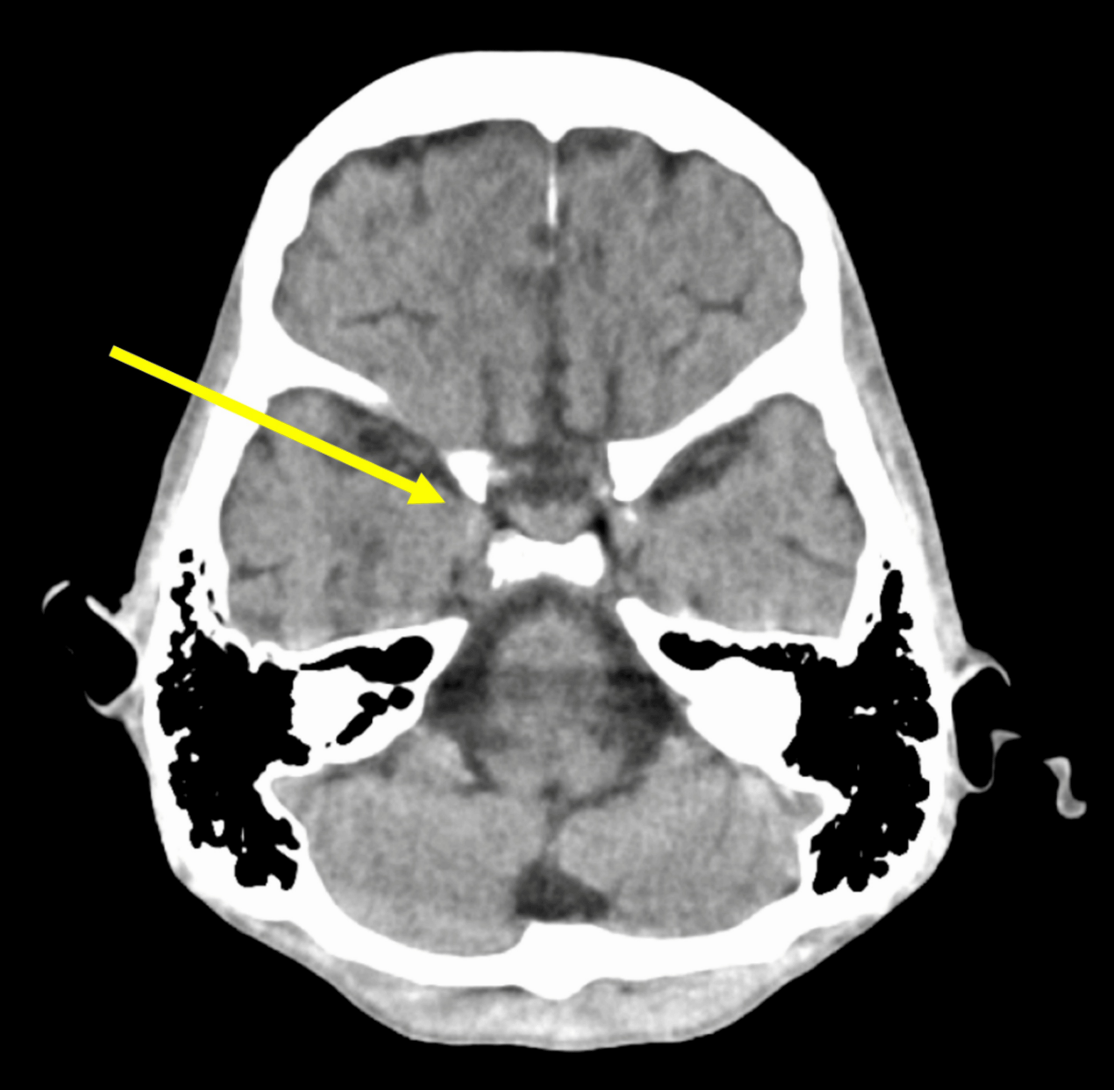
CT of the head shows no significant findings, including the pituitary gland.
